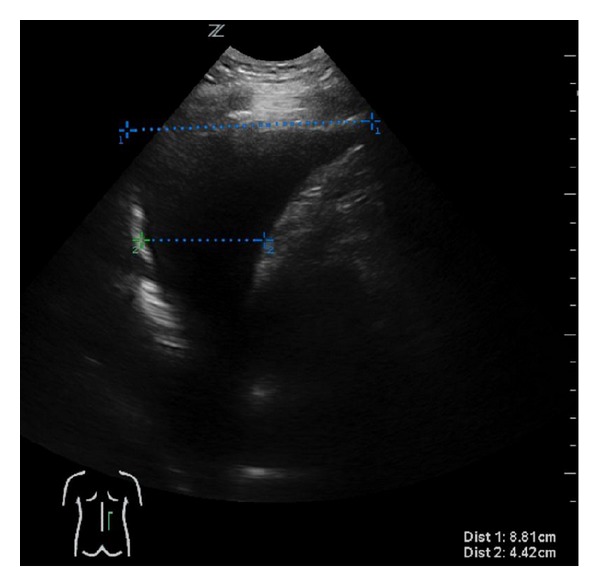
Ultrasound of lower thorax.
